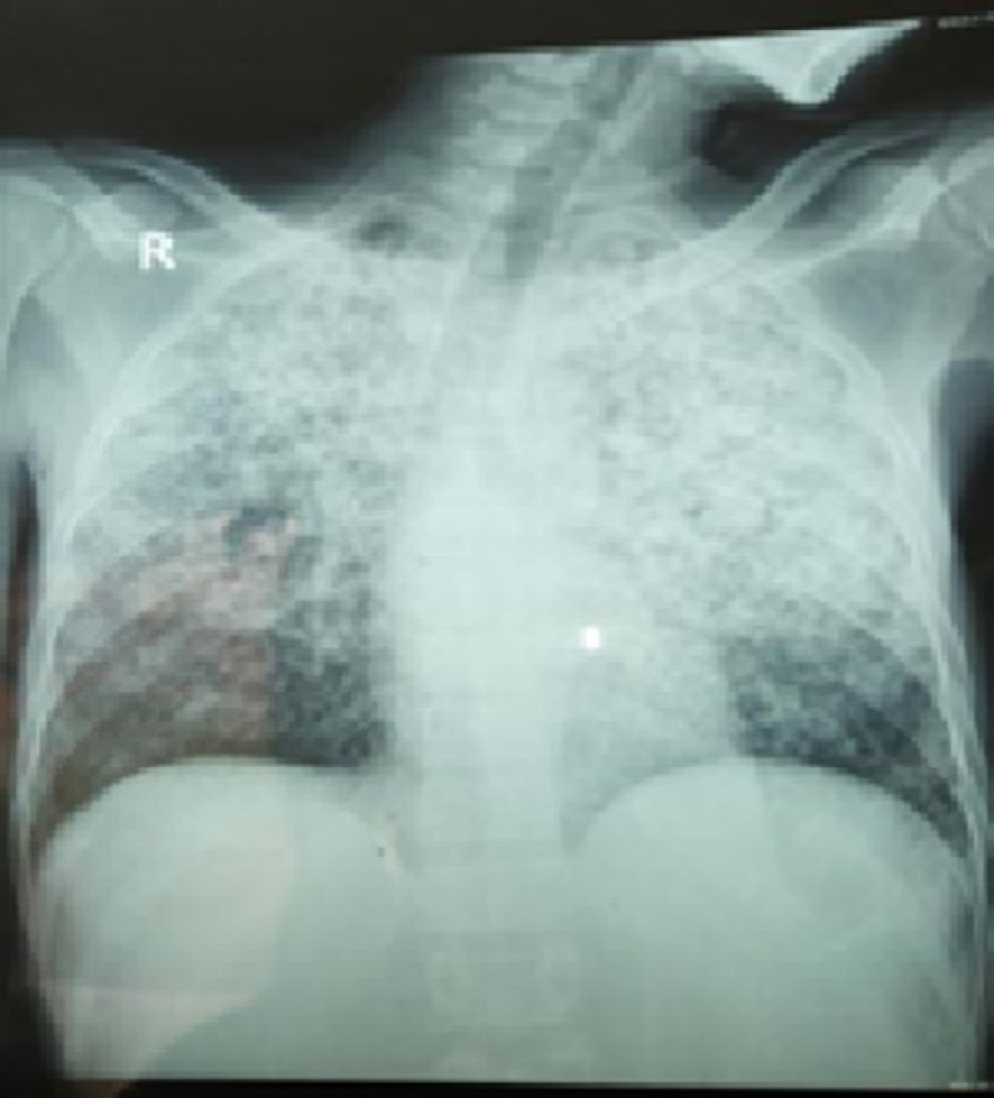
Postero‐anterior chest radiograph showing military‐like opacities on both lungs with relative sparing of lung bases.
